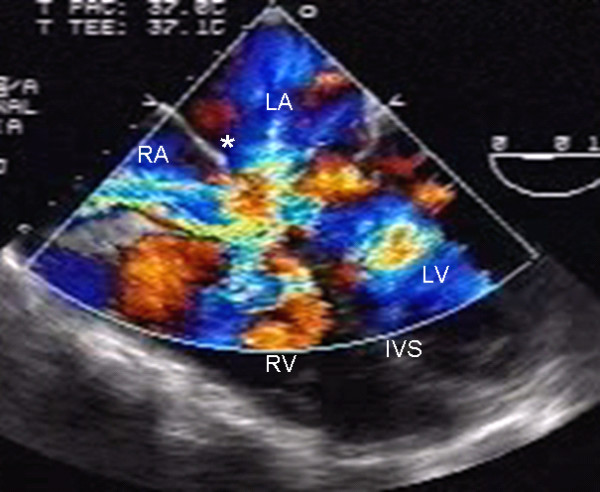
Two-dimensional transesophageal echocardiogram at 0° with color Doppler shows the shunt above the valve plane. Note the interatrial septum (asterisk). Abbreviations as before.
